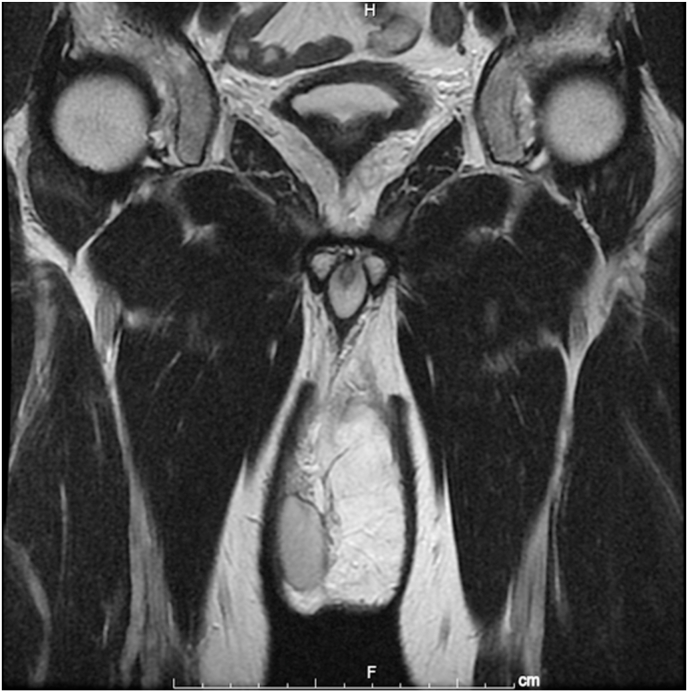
Pelvis MRI: T2-weighted coronal image.
